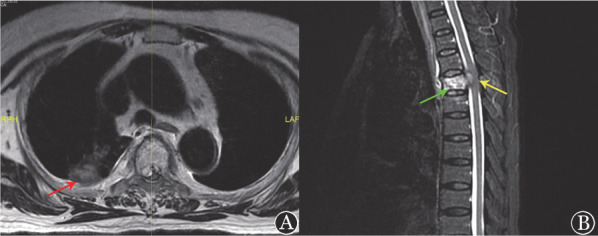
弥漫大B细胞淋巴瘤患者（例3）发生侵袭性真菌病的椎体增强核磁共振（MRI）表现 A 胸椎MRI轴位T2，右肺上叶后段胸膜下团块状长T2信号（红色箭头）；B 胸椎MRI压脂矢状位，第4胸椎椎体、附件及椎管内可见短T1、长短混杂T2信号（绿色箭头），继发相应节段椎管狭窄（黄色箭头），脊髓受压向后移位
